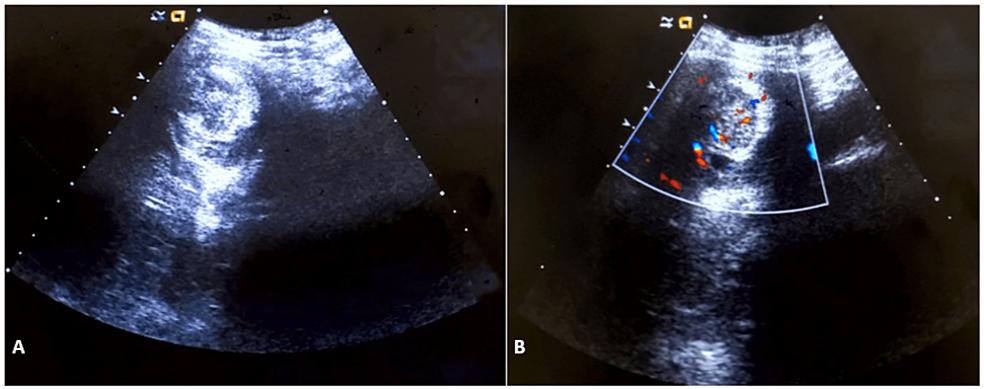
Intussusception - image 1 A. Cross-sectional 1-4 MHz grayscale ultrasound image obtained over the right lumbar region demonstrates the target sign (anteroposterior diameter of 5.41 cm). B. Target sign with Doppler
